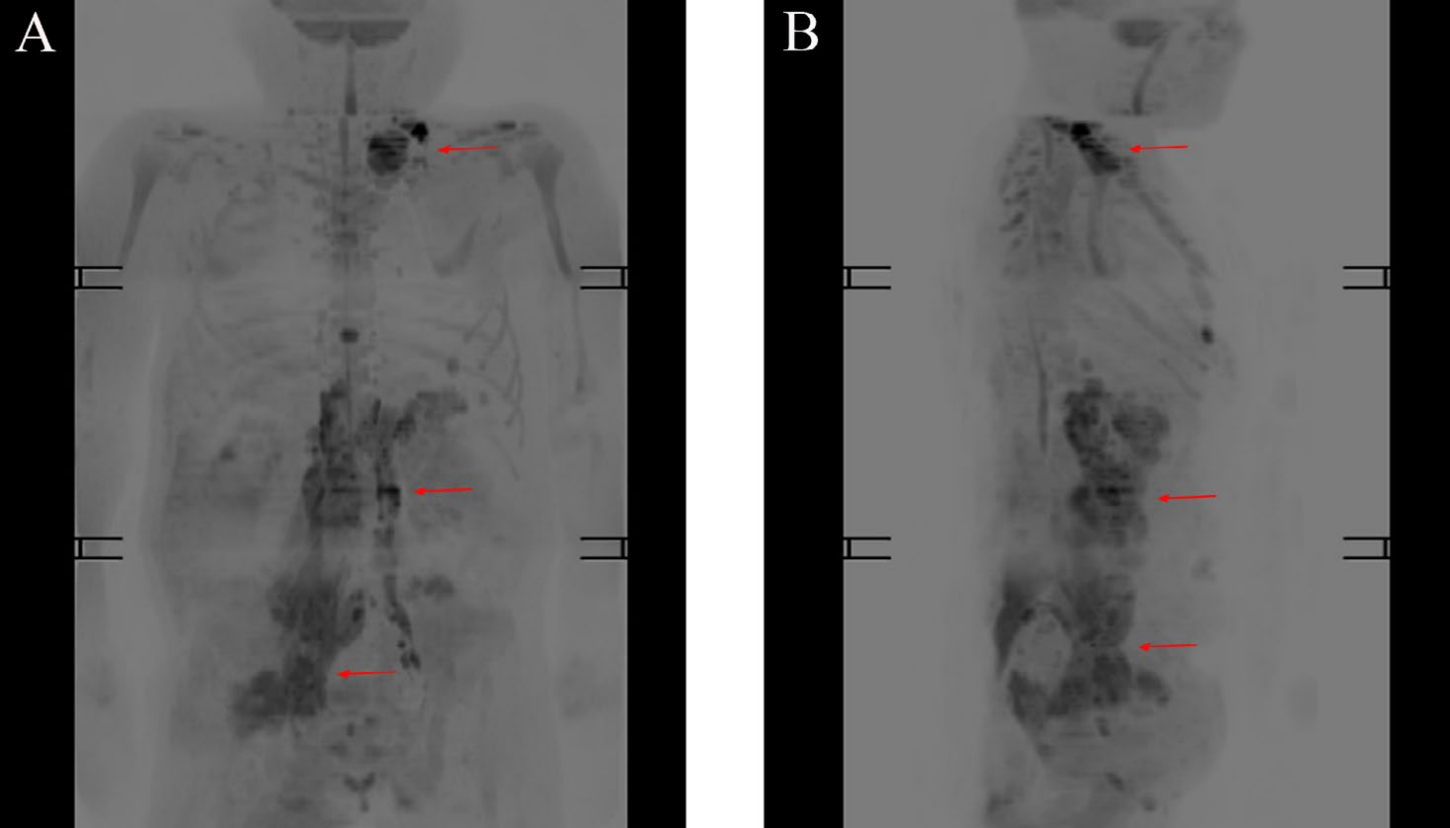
Whole‐body magnetic resonance imaging (MRI) with diffusion‐weighted imaging (DWI) shows multiple enlarged lymph nodes (arrows) in the left neck base, left upper and lower clavicle regions, abdominal cavity, retroperitoneum, and right pelvic wall. It indicated that lymphoma had invaded the stomach, bladder, right gluteus maximus, and lumbosacral eriospinal muscle.
